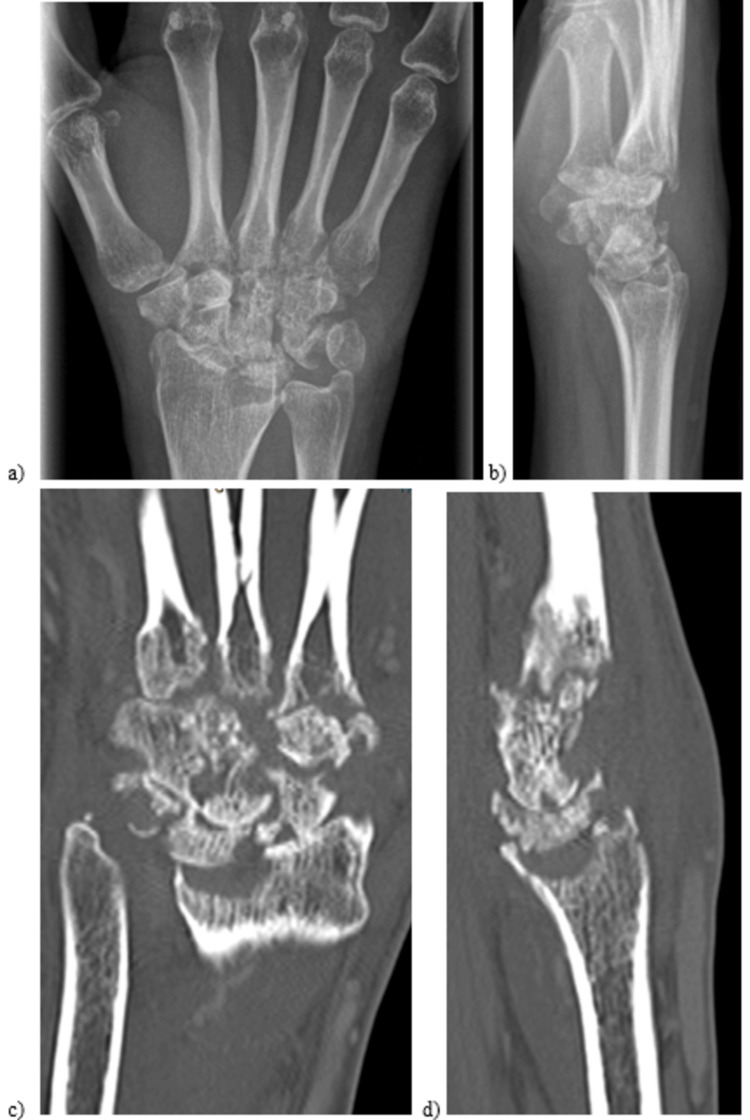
Repeat imaging obtained two months following the initial onset of symptoms demonstrating erosive damage and diffuse destruction of the carpus, radiocarpal joint, and carpometacarpal joints, with carpal subluxation into the eroded radius. Right wrist radiograph: a) anteroposterior view and b) lateral view. Computed tomography: c) coronal view and d) sagittal view.
